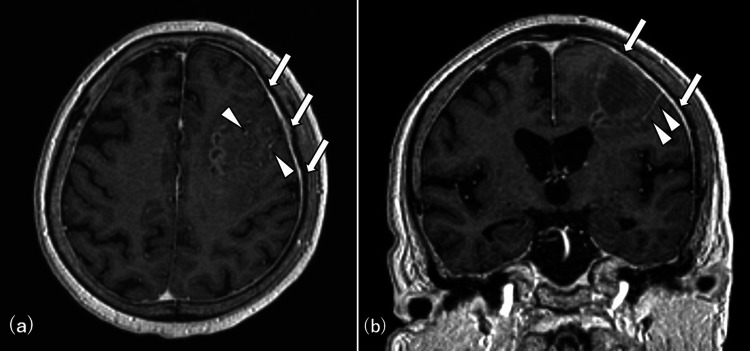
MRI showed meningeal enhancement (arrows) and vascular wall thickening (arrowheads) on gadolinium-enhanced T1-weighted imaging MRI: magnetic resonance imaging (a) Axial image. (b) Coronal image
